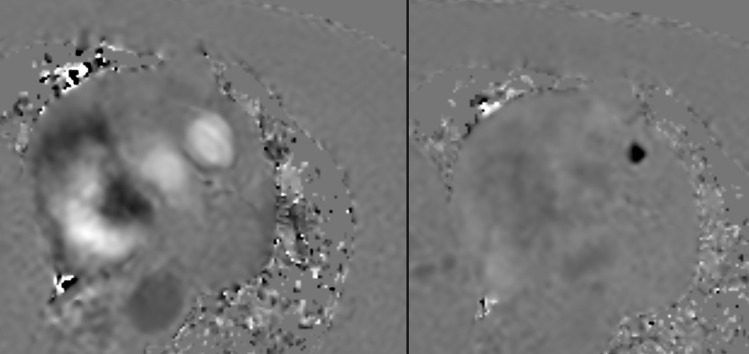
Pulmonary valve regurgitation of 30%: axial phase‑contrast (Q‑flow) cardiac MRI showing retrograde flow into the right ventricle during diastole.
